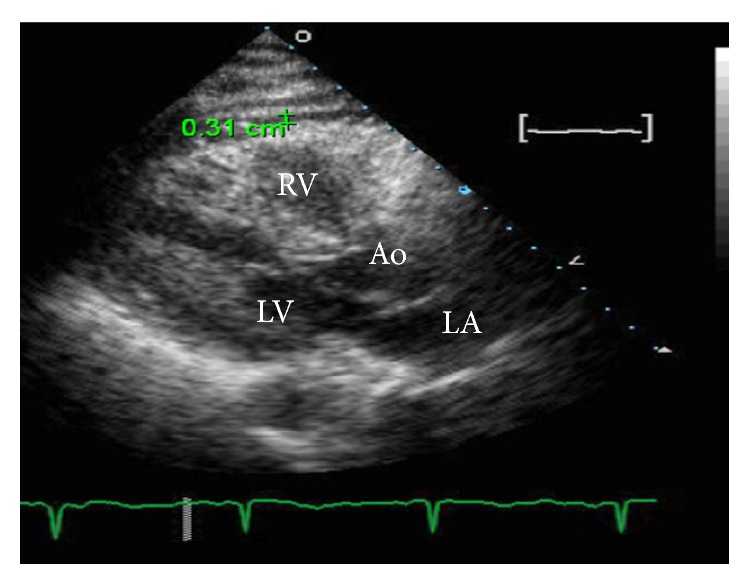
Echocardiographic evidence of pericardial effusion (measuring 0.31 cm) after aortic dissection on parasternal long axis view. Ao = aorta, RV = right ventricle, LV = left ventricle, and LA = left atrium.
